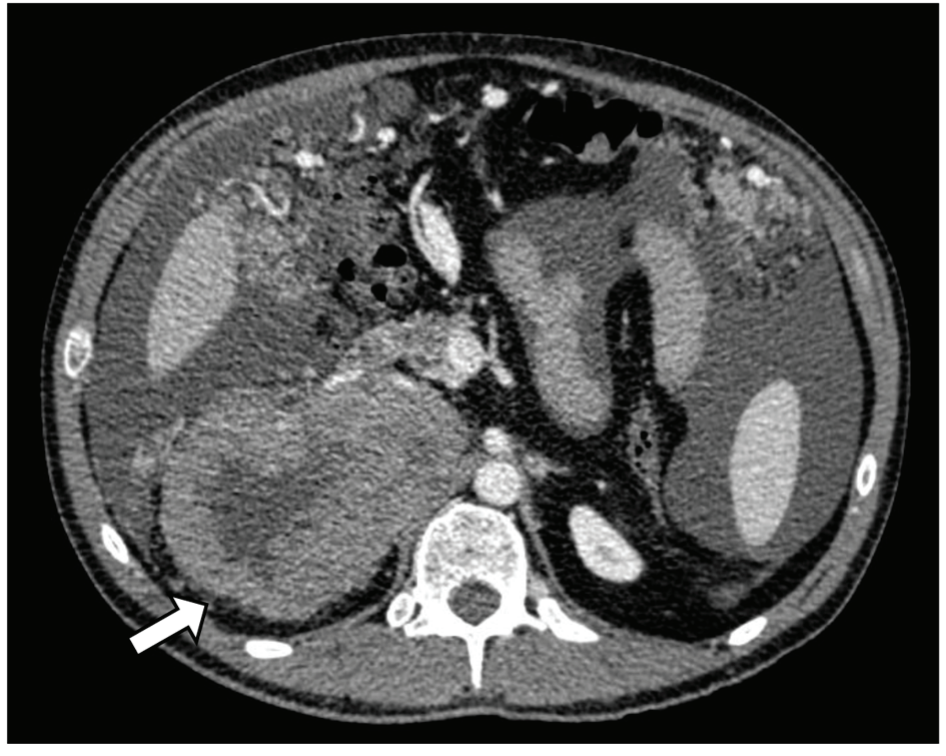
CT scan of adrenocortical carcinoma showing necrotic right adrenal mass in close proximity to the body/tail of pancreas and third portion of the duodenum (white arrow).
